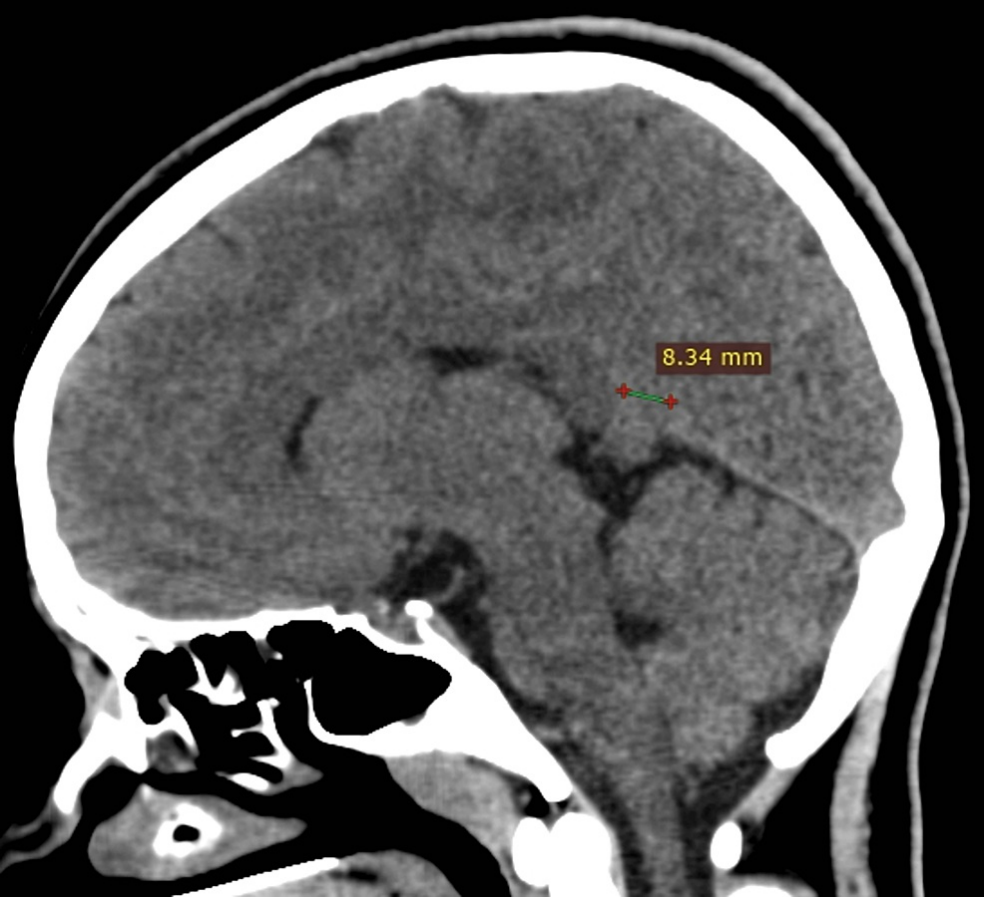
CT, brain window, right paramedian sagittal section. A small (8 mm) defect at the anterior edge of the tentorium, allowing herniation of a small part of the occipital lobe into the cistern below. CT: computed tomography
